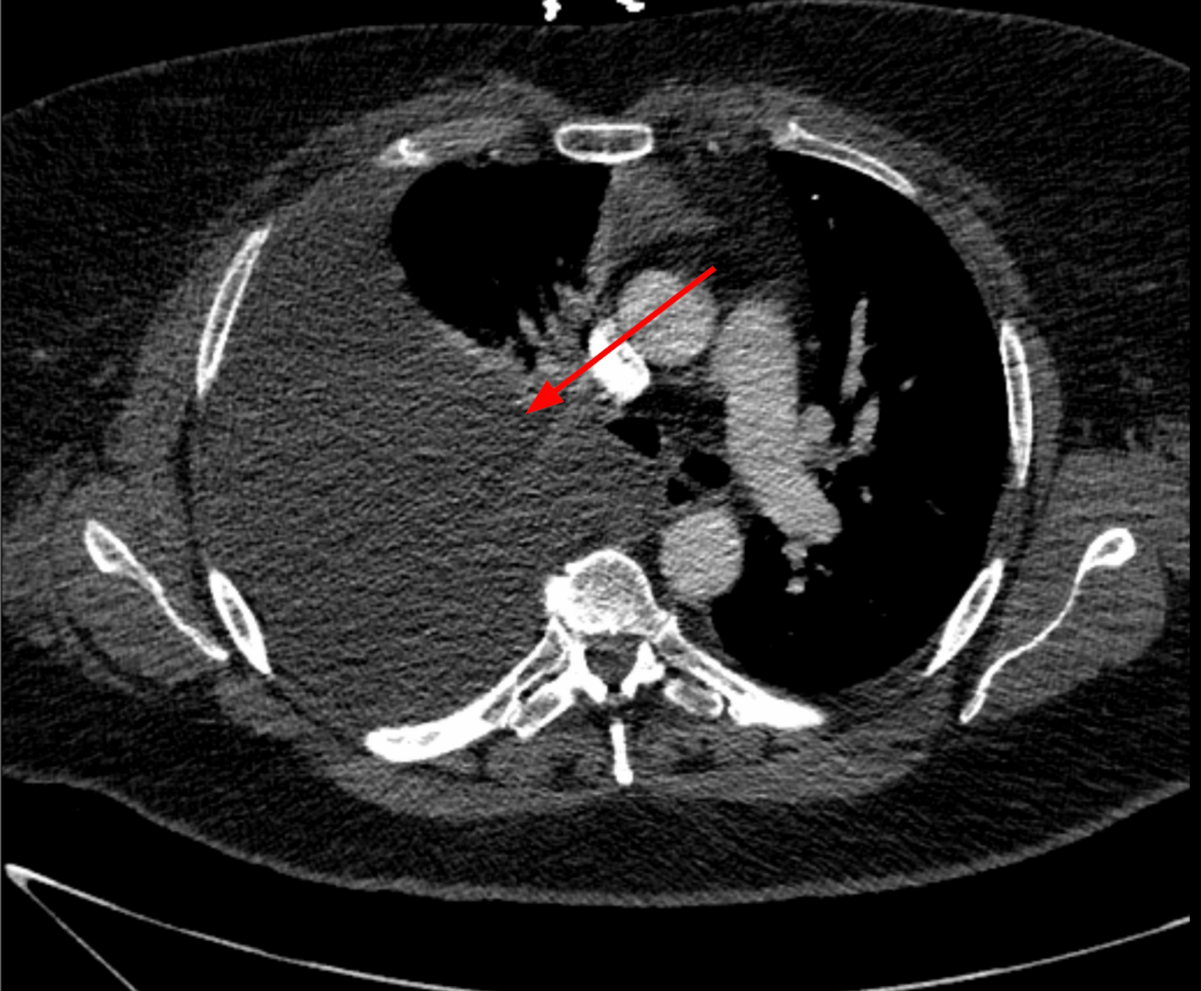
CT chest angio showing a large right pleural effusion. Large right pleural effusion.
